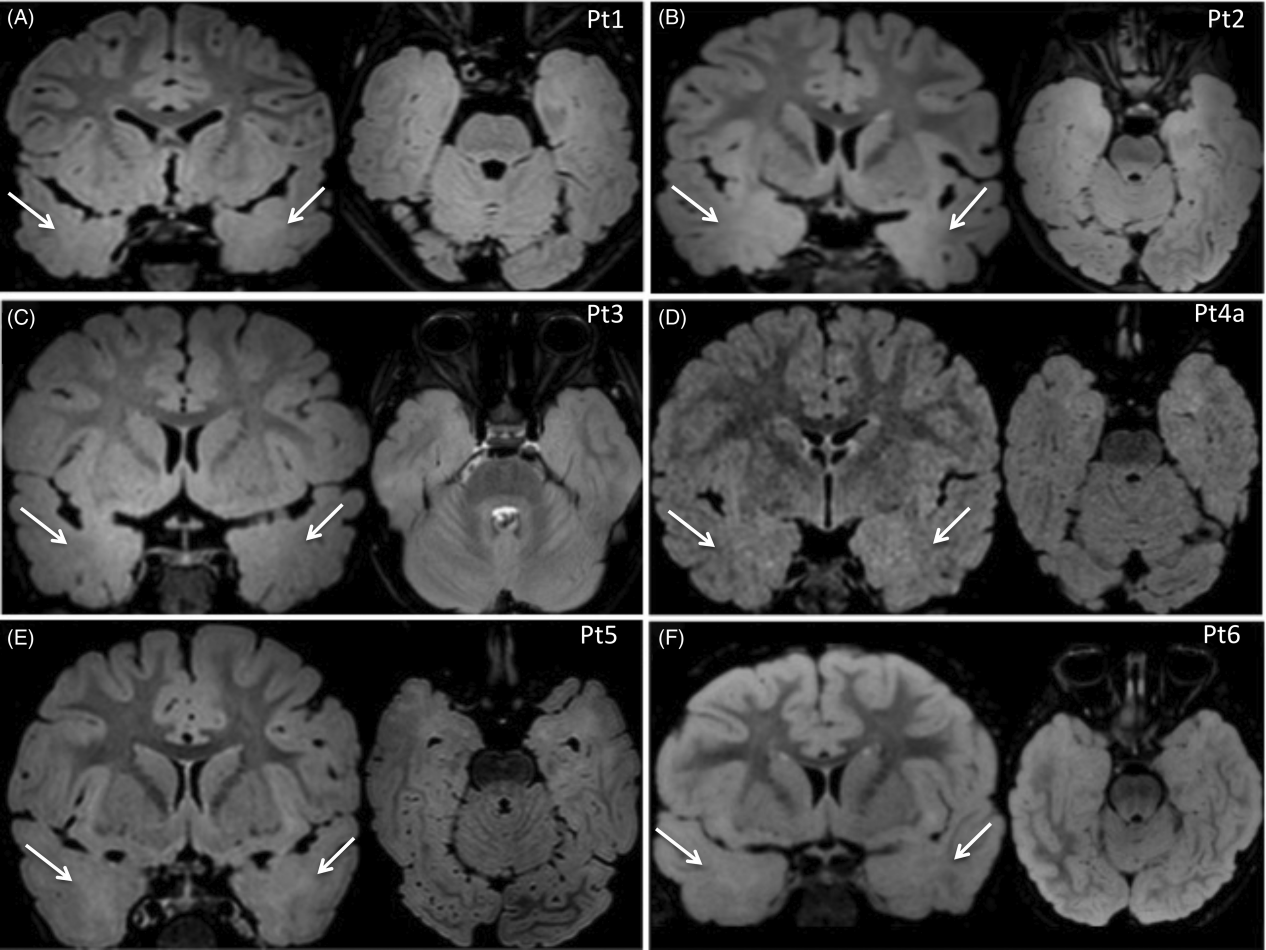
A, MRI of Patient 1 (3 y): T2‐weighted FLAIR coronal and axial sections show bilateral temporal gray/white matter (GM/WM) blurring (white arrows). B, MRI of Patient 2 (3 y): T2‐weighted FLAIR coronal and axial sections show bilateral temporal GM/WM blurring (white arrows). C, MRI of Patient 3 (9 y): T2‐weighted FLAIR coronal and axial sections show bilateral temporal GM/WM blurring (indicated by white arrows). D, MRI of Patient 4a (2 y): T2‐weighted FLAIR sections show coronal and axial bilateral temporal GM/WM blurring and WM signal hyperintensity (indicated by white arrows). E, MRI of Patient 5 (2 y): T2‐weighted FLAIR coronal and axial sections show GM/WM blurring and WM signal hyperintensity (white arrows). F, MRI of patient 6 (5 y): T2‐weighted FLAIR coronal and axial sections show bilateral temporal GM/WM blurring and WM signal hyperintensity (white arrows). All MRI images were performed at 3T.
